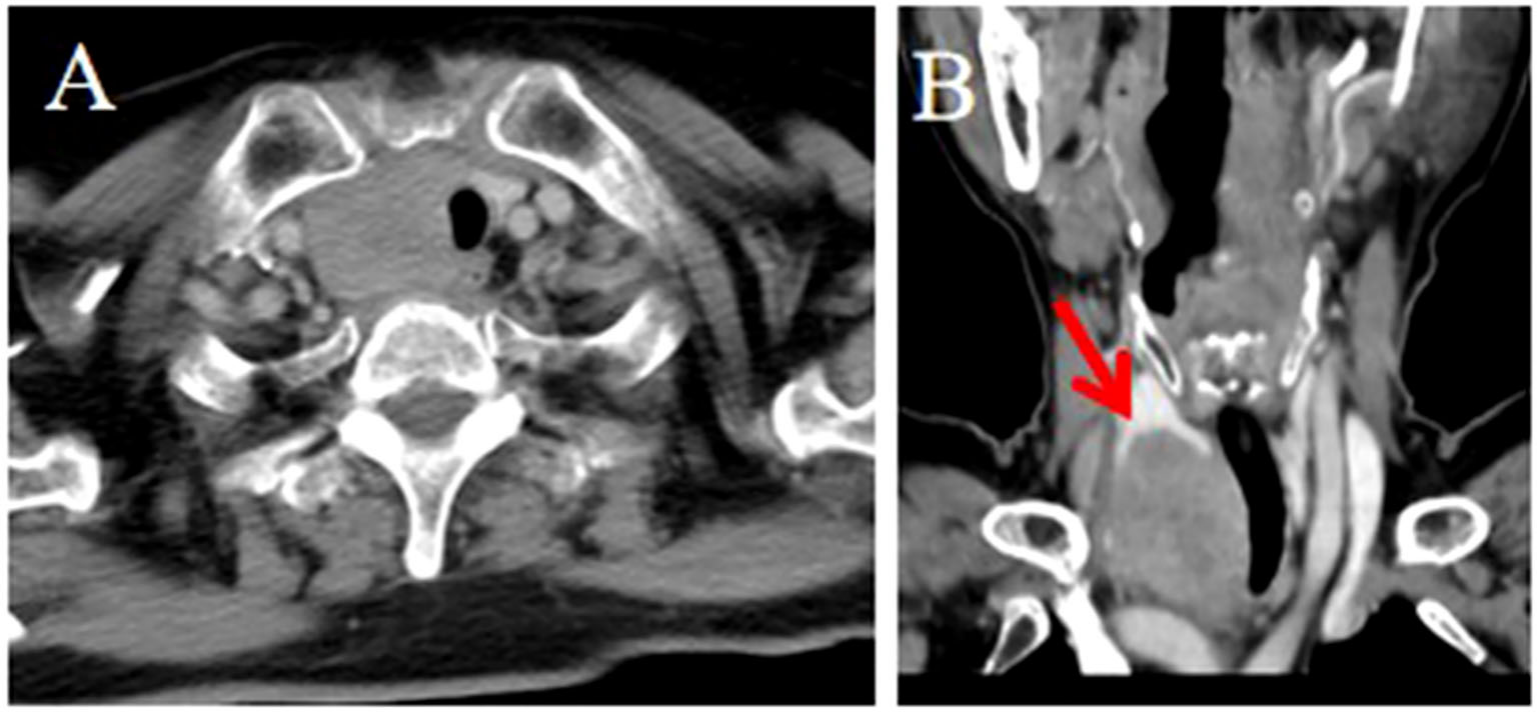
CT imaging of the ITTC. (A, B) Mass demonstrating homogeneous enhancement at the right tracheoesophageal groove with extension to the thyroid’s lower pole; (B) The tumor–thyroid interface formed an arc-shaped boundary (red arrow) at multiplanar reconstruction, involved the esophagus, causing tracheal stenosis, which was confirmed by surgery.
